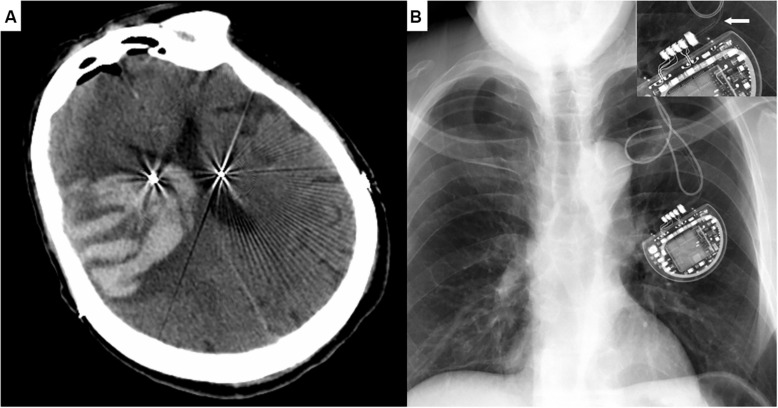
Representative Cases. (A) Cranial CT image of Patient #7 demonstrating the massive intracranial hematoma three days after the surgery, with symptoms of a generalized seizure. (B) Chest X-ray image of Patient #36 showing the fracture of extension wire near the IPG. Enlarged damaged wire in the right upper corner.
